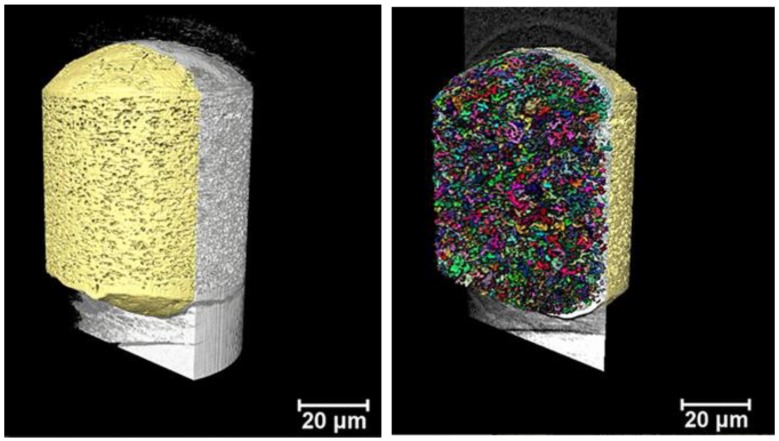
Volumetric representation the inner part of one reconstructed microsphere of Risperdal Consta^®^ imaged with nano-CT. The left picture shows the X-ray signal in gray and the microsphere PLGA matrix pseudocolored in yellow. On the right, the small colored structures represent the dispersed internal spherical structures (ISS), which can be attributed to the dispersed drug particles. The volume of the ISS is about 13% of the total volume of the particle.
